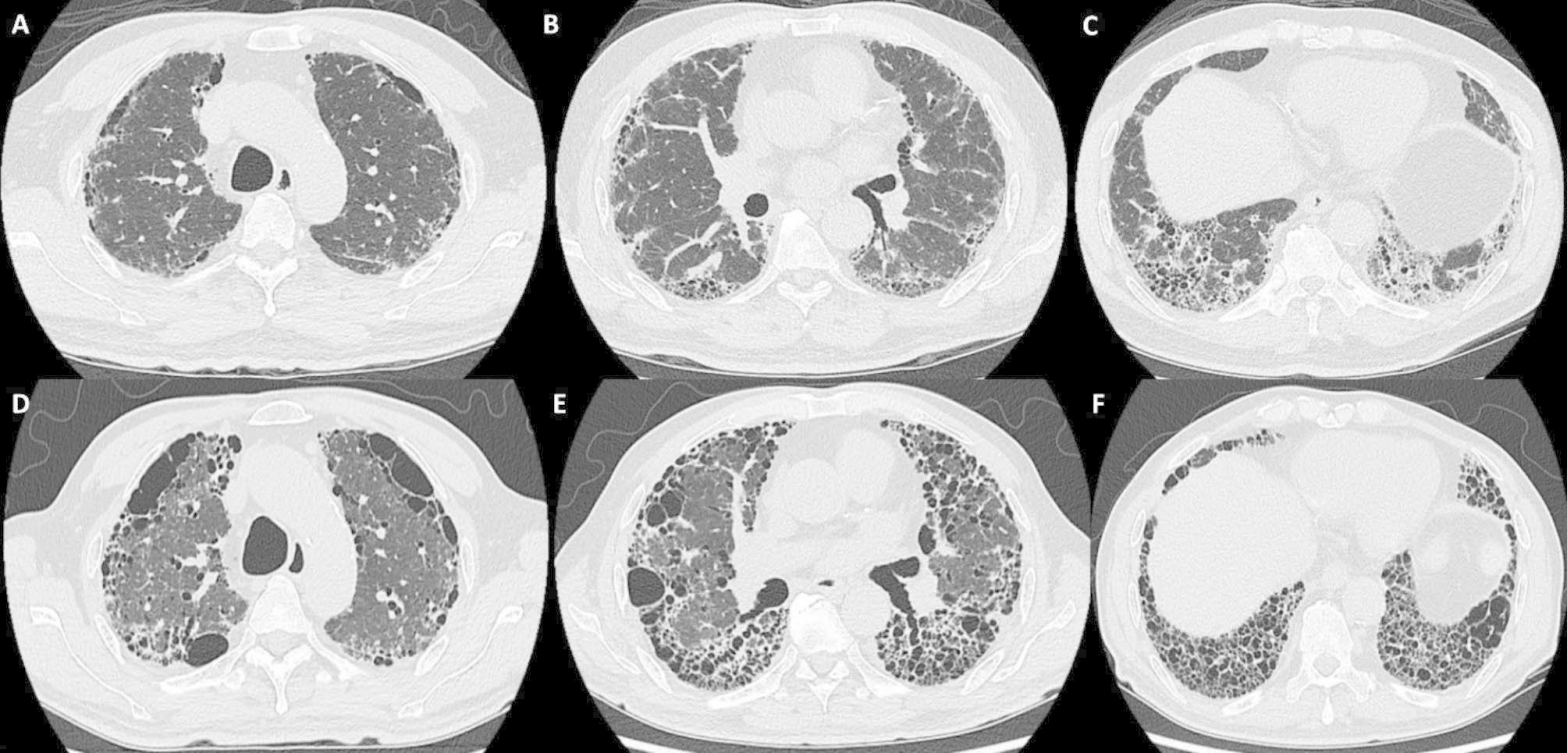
High resolution computed tomography imaging of the chest in a patient with Sjögren’s syndrome-associated interstitial lung disease (PF-ILD). Serial axial images at baseline (Panel A-C) and at 24 months (Panel D-F)
